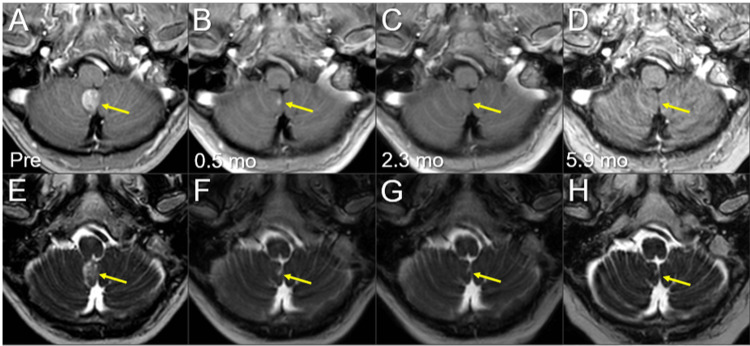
Magnetic resonance images before and after the third SRS of the right cerebellar new lesion. The images show (A-D) axial CE-T1-WIs and (E-H) axial T2-WIs (A, E) five days before (Pre) the third SRS (17.3 mos after the initiation of CRT); (B, F) at 0.5 mo after the initiation of the third SRS; (C, G) at 2.3 mos; and (D, H) at 5.9 mos (23.9 mos after CRT). (A-P) These images are shown at the same magnification and coordinates under co-registration and fusions. (A-H) The solid enhancing lesion (arrows in A and E) shrank remarkably at 0.5 mo (arrows in B, F) and disappeared, only leaving the faint scar (arrow in G), at 2.3 mos (arrows in C and G). The lesion remained completely regressed without any adverse radiation effects at 5.9 mos (arrows in D and H). CE, contrast-enhanced; WIs, weighted images; SRS, stereotactic radiosurgery; CRT, chemoradiotherapy; mo, month
